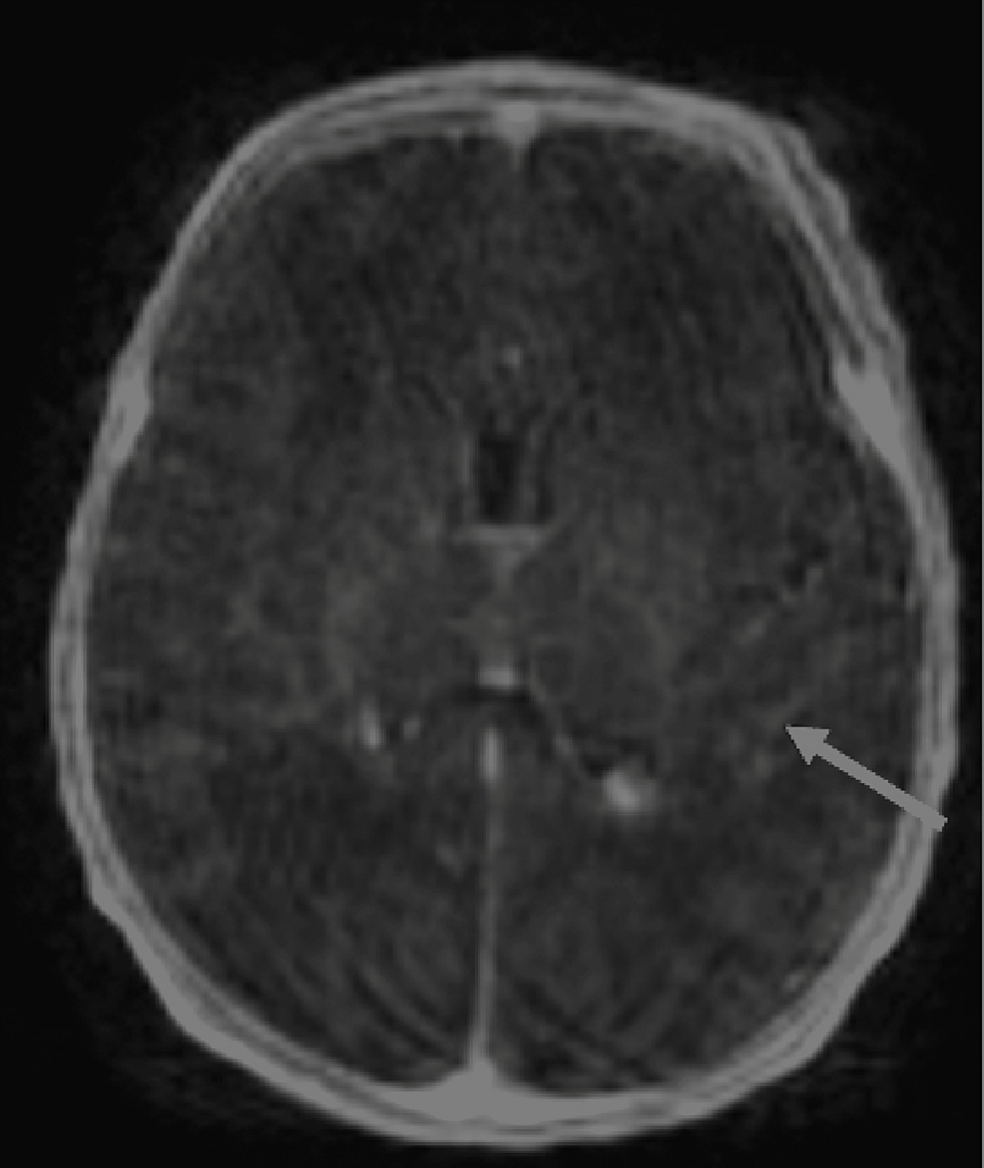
Contrast-enhanced T1-weighted axial MRI image of the brain shows leptomeningeal enhancement (arrow).
